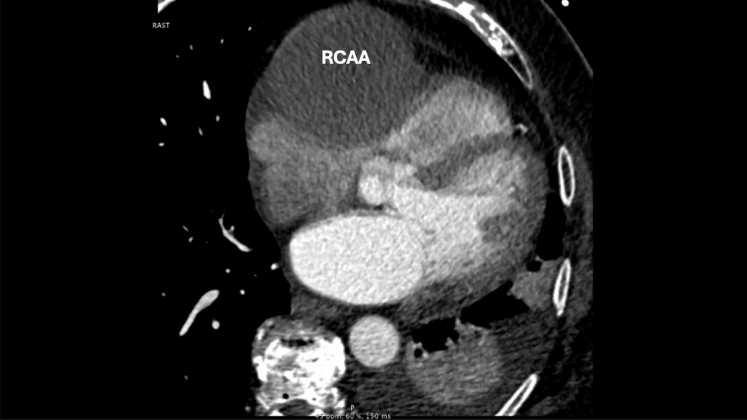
Computed tomography angiography demonstrating a right coronary artery aneurysm (RCAA).
